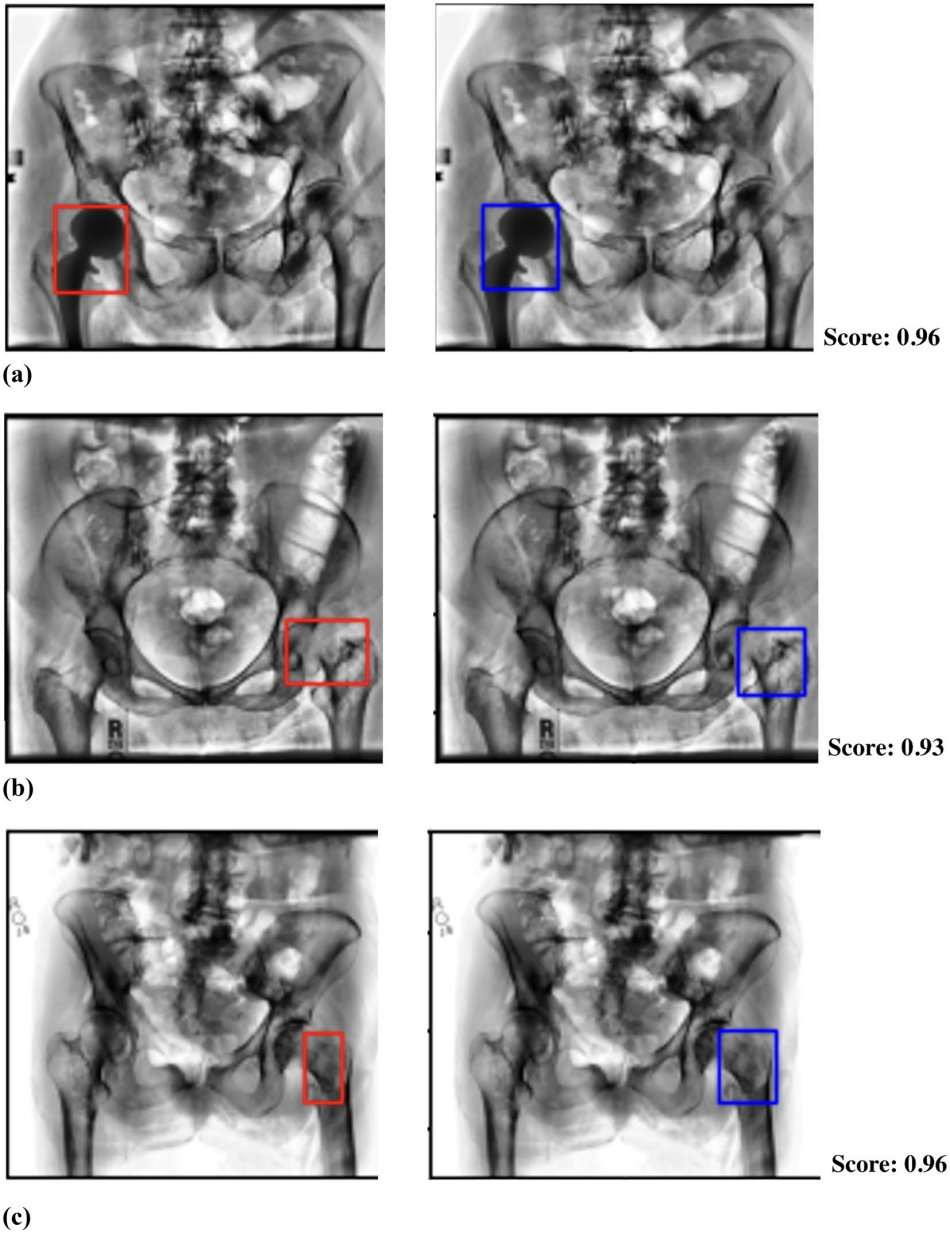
Example visualizations of ground-truth fracture bounding boxes (left) vs. predicted fracture bounding boxes by VarifocalNet (right). Images are radiographs preprocessed via CLAHE, as described in Section "Data preprocessing".
